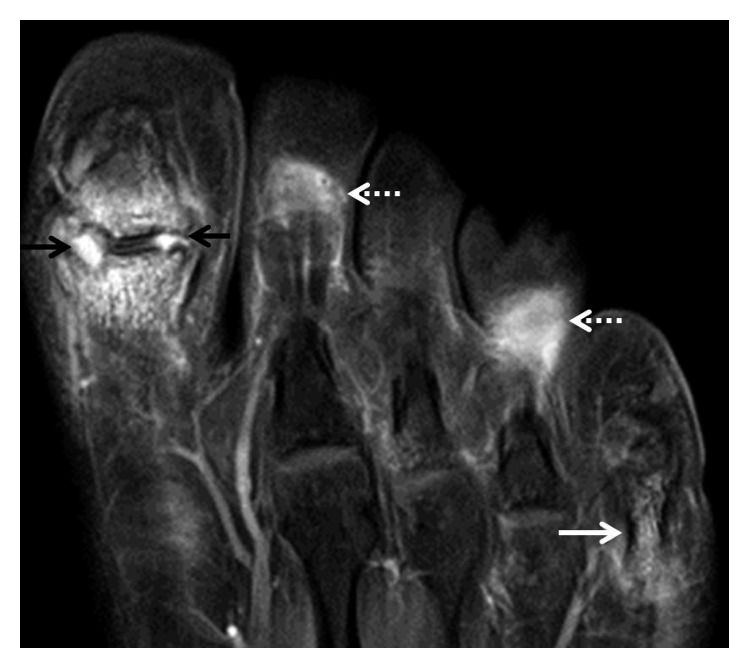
Axial T1 fat-saturated magnetic resonance imaging (MRI) of the foot obtained with gadolinium contrast administration demonstrated marginal erosions at the great toe interphalangeal joint, associated synovitis, and bone marrow enhancement (black arrows). Erosive changes involving the fifth metatarsophalangeal joint were present with bone marrow enhancement in the proximal phalanx (solid white arrow). Enhancing inflammatory changes in the second and fourth toe plantar soft tissues were present (dashed white arrows).
